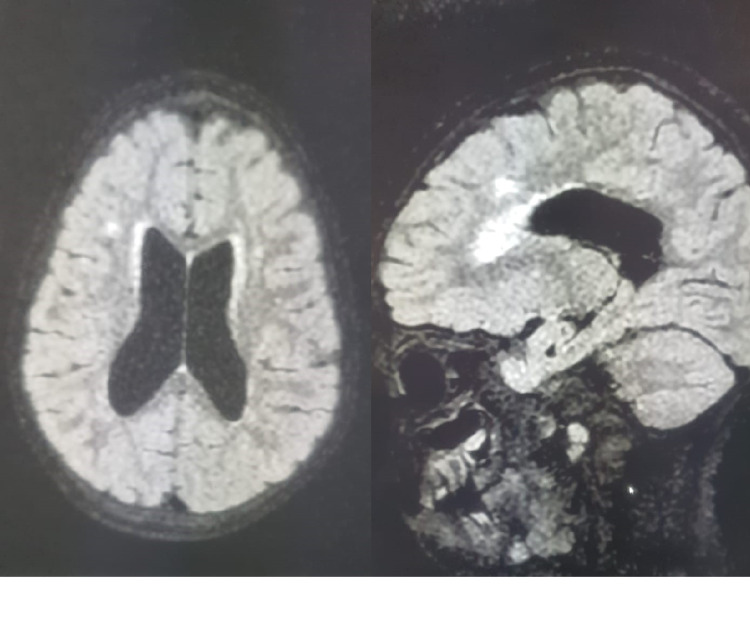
Brain MRI showing moderate leukodystrophy.
